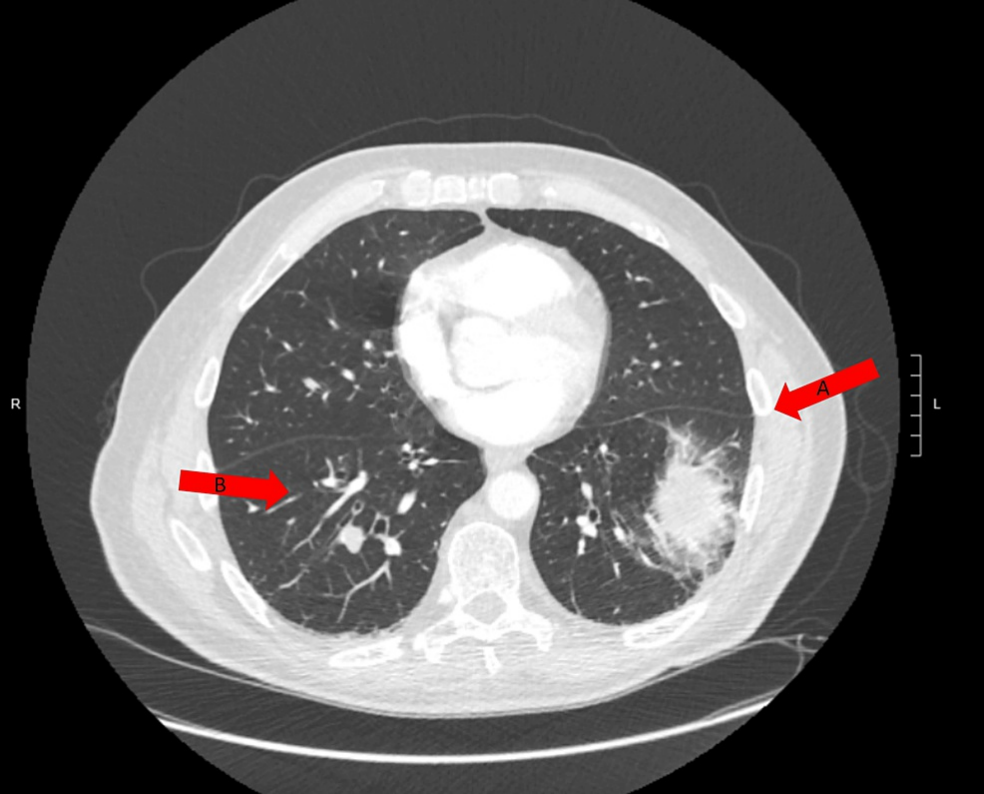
CT chest PE protocol showing left lower lobe pneumonia (arrow A), and multiple filling defects in the pulmonary arteries in the right lower lobe of the lung (arrow B) PE - pulmonary embolism
